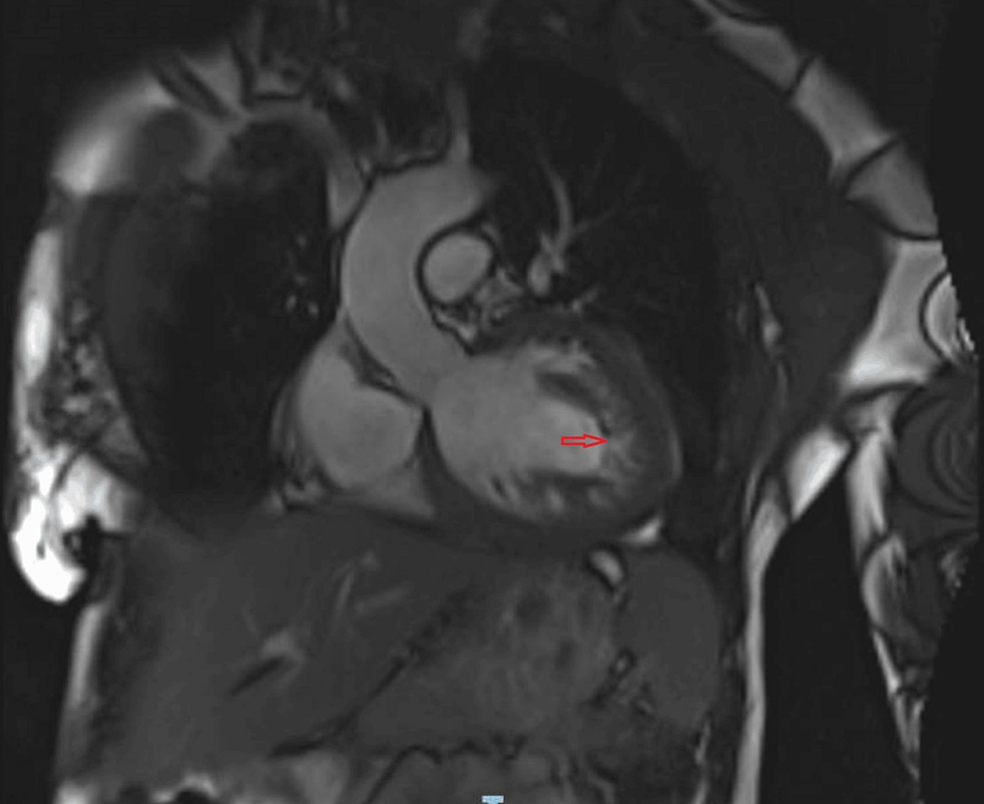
Cardiac MRI showing increased left ventricular thickness. MRI: magnetic resonance imaging
